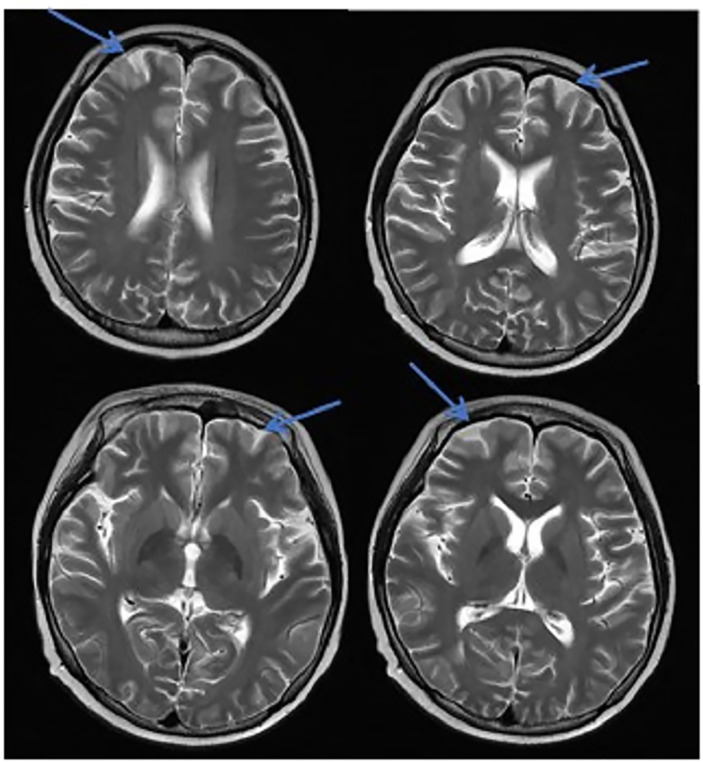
MRI in patients with CNS infection. The CNS infection showed flair hyperintensity in the cerebral cortex, but no clear display on the T2WI, T1WI, DWI, and no enhancement after enhancement.
